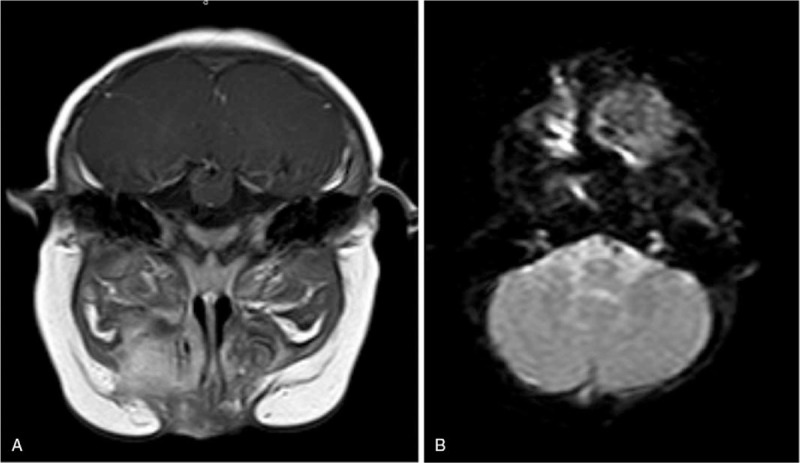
The nasopharynx MRI showed the presence of a new mass of abnormal signal in the same region as before, with a size of 28.8 mm × 28.3 mm × 11.6 mm.
